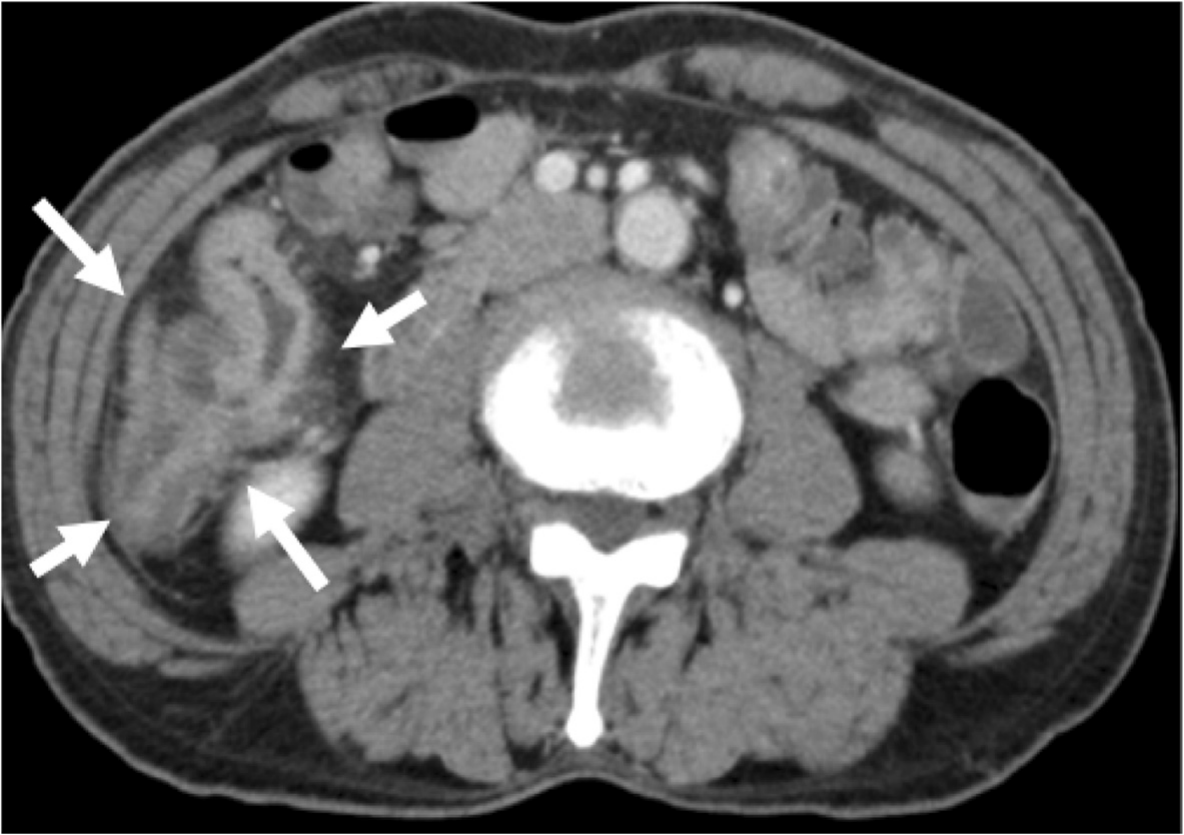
Abdominal enhanced computed tomography showed a sigmoid colostomy (because of Miles operation), a tumor located in the hepatic flexure (*white arrows* show), and lymph node metastasis. However, distant metastasis was not observed
